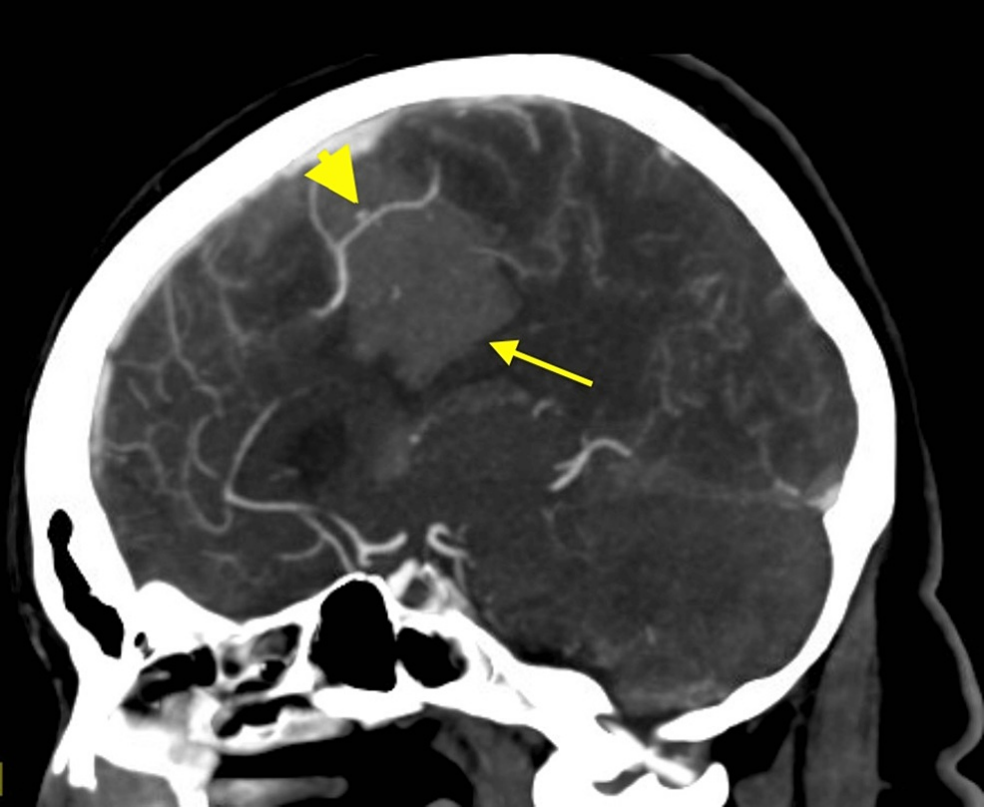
CTA of the head and neck showing ruptured pseudoaneurysm. A sagittal view of CTA of the head and neck on the arterial phase demonstrates a 2 mm ruptured pseudoaneurysm (arrowhead) within a left frontal parenchymal hematoma (arrow). CTA, computed tomography angiography
